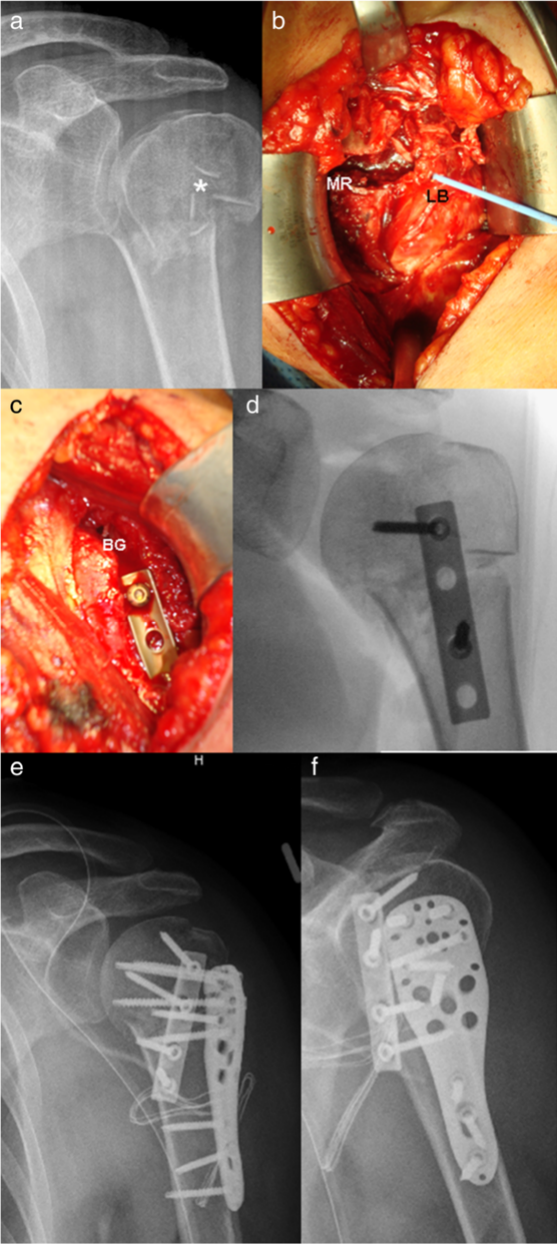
a a.p. view of a proximal humerus fracture with metaphyseal comminution (*) b intraoperative view showing the comminuted zone; long biceps tendon with loop (LB), metaphyseal region (MR) c, d the reduction is accomplished with the inverted tubular plate within the sulcus of the biceps tendon; bicipital groove (BG) e, f postoperative a.p. and y- radiograph with double plate configuration
